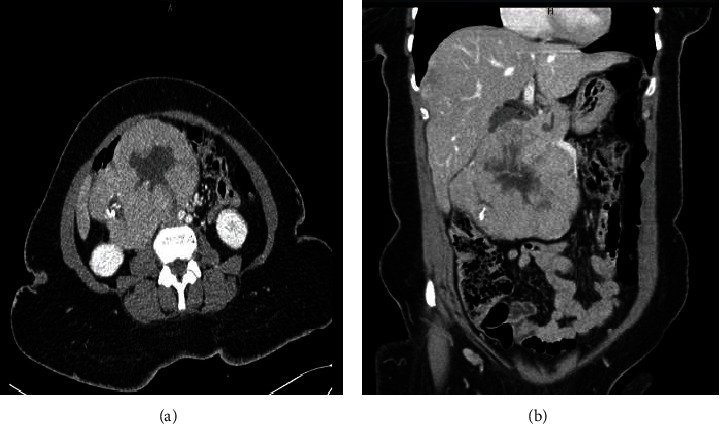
The postcontrast CT scan images in two orientations: (a) shows the axial view, while (b) depicts the coronal view.
